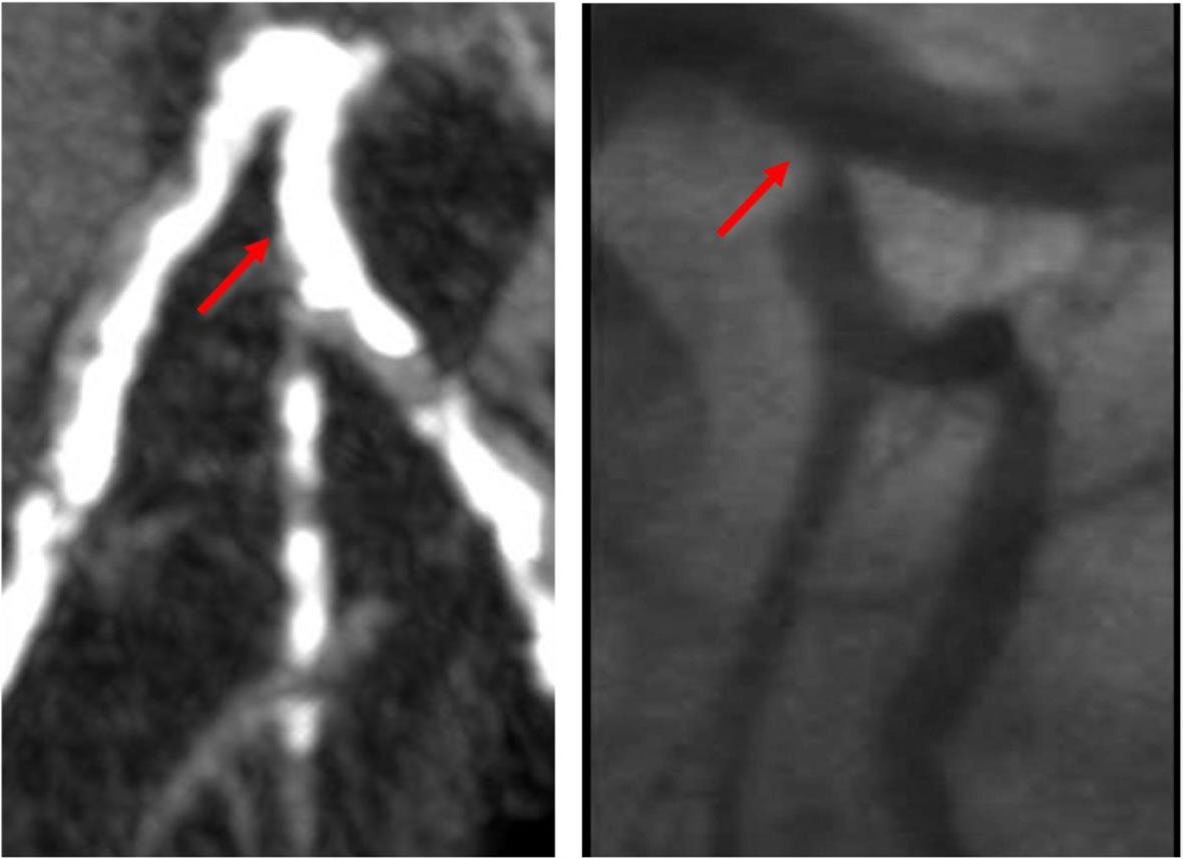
In this example, the proximal part of the left circumflex coronary artery (LCx) has heavy uniform calcification, resulting in blooming in the CT image. This results in the inability to distinguish the severe ostial LCx stenosis (red arrow), that is easily identified using ICA
